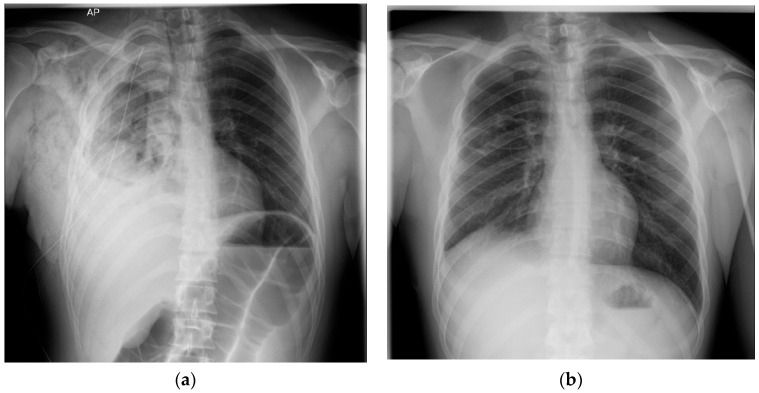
Post-surgery radiological evaluation. (a) Chest-X-ray the third day after surgery shows multiple heterogenous opacities occupying two-thirds of the right pleural cavity (right intrapleural hematoma), an extrapleural right hematoma, and a large bowel distension. (b) Chest-X-ray two-weeks after discharge from Thoracic Surgery Department showing lung re-expansion and a medium-intensity opacity occupying the right costo-diaphragmatic recess.
